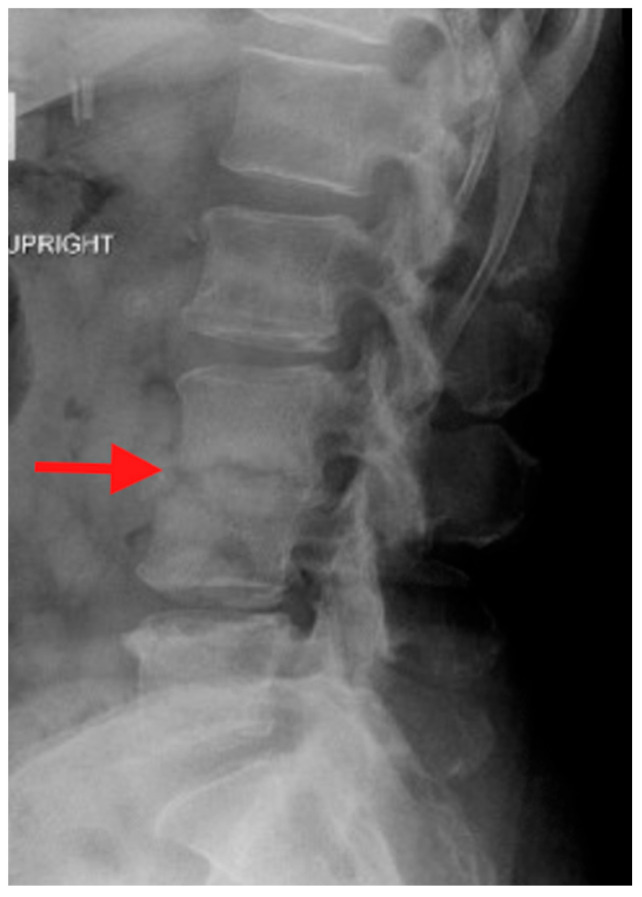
Upright lateral plain radiograph of the lumbar spine. The red arrow is pointing to the L3–4 disc space. Irregularity of the vertebral endplates and collapse into a kyphotic deformity can be seen.
